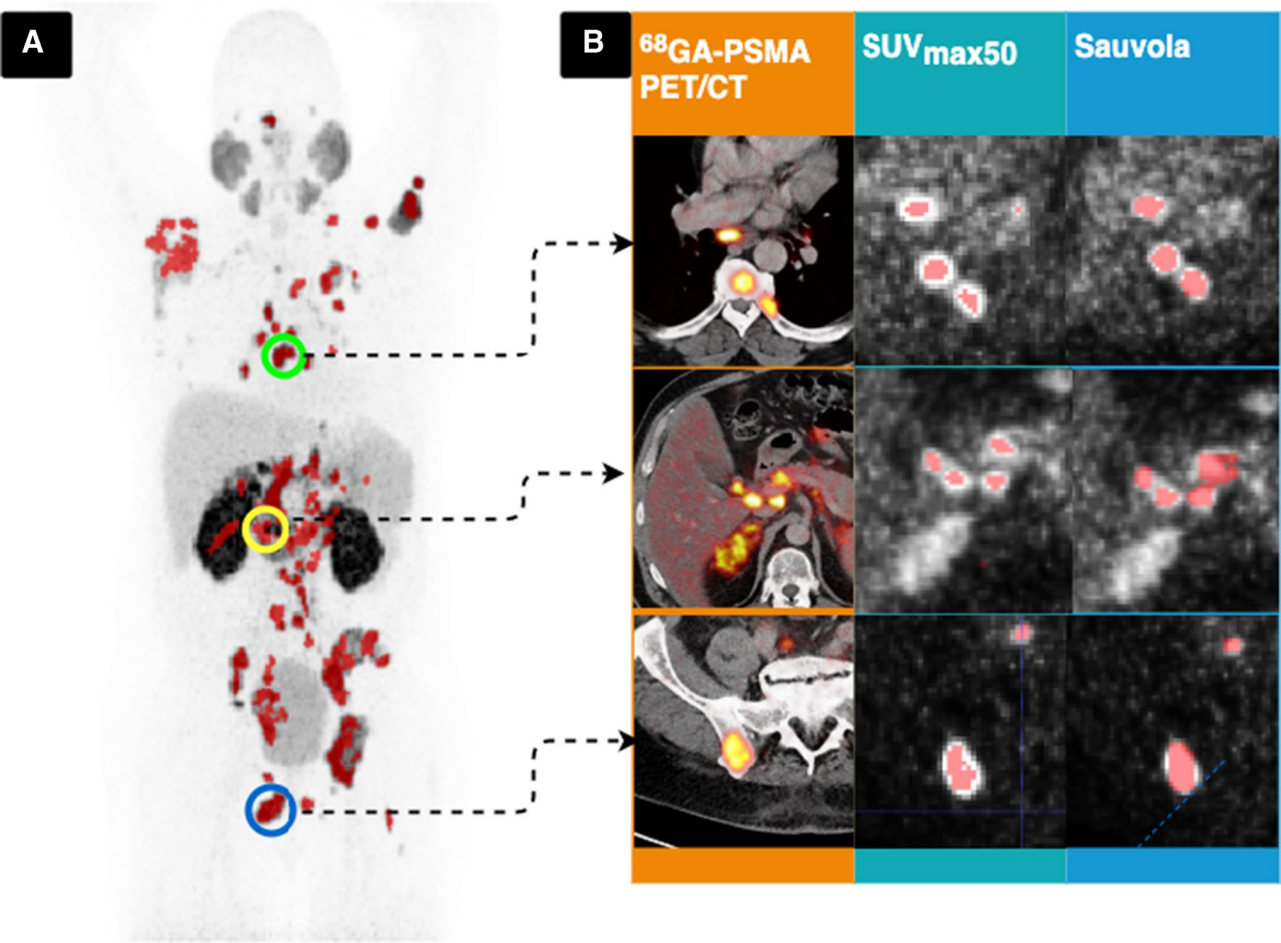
A, Maximum intensity projection of PSMA PET with overlaid thresholding binary mask of SUVmax50 for visual control. Green circle: subcarinal lymph node metastasis in the mediastinum and bone metastases in thoracic vertebrae and ribs; yellow circle: diffuse intra-abdominal lymph node metastasis at the hepatic hilum; blue circle: bone metastasis in the right ilium. B, Example comparison of PSMA PET positive lesions using the binary mask of SUVmax50 and Sauvola thresholding method
